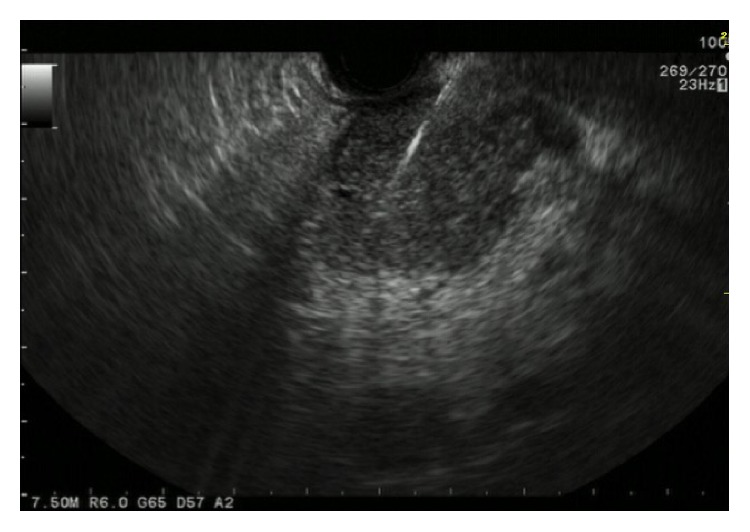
Endoscopic ultrasound findings. A dumbbell-shaped mass was present in the body of the pancreas.
